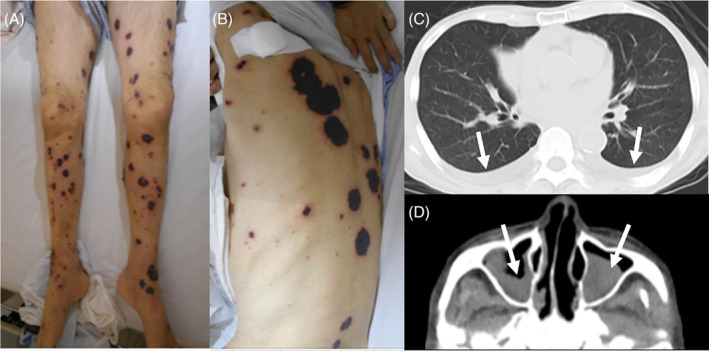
Findings of our case on admission. (A,B) The patient presented with numerous purpuric lesions accompanied by erythema and hematomas distributed on the extremities and trunk. (C) Bilateral pleural effusion was observed on chest CT. No other significant findings were noted (white arrows). (D) Head CT revealed mucosal thickening in the bilateral frontal sinuses, ethmoidal sinuses, maxillary sinuses, and sphenoid sinuses (white arrows).
